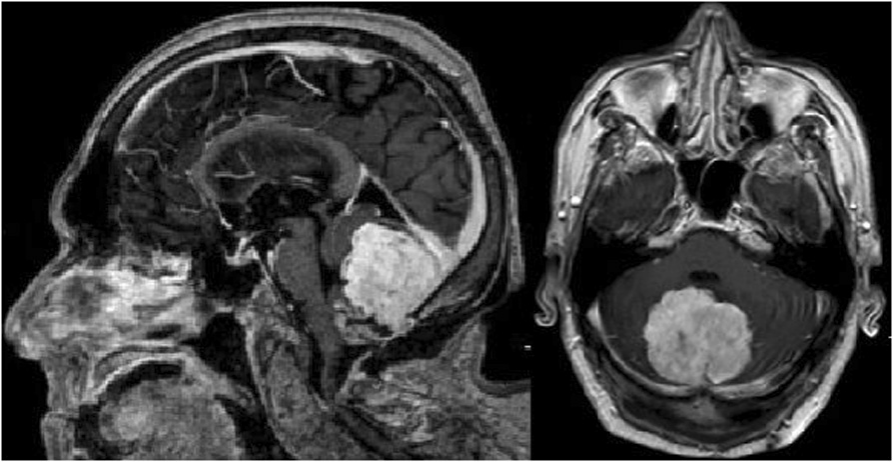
At 6-months follow-up, a magnetic resonance imaging showed an early regrowth of the fourth ventricle tumor
